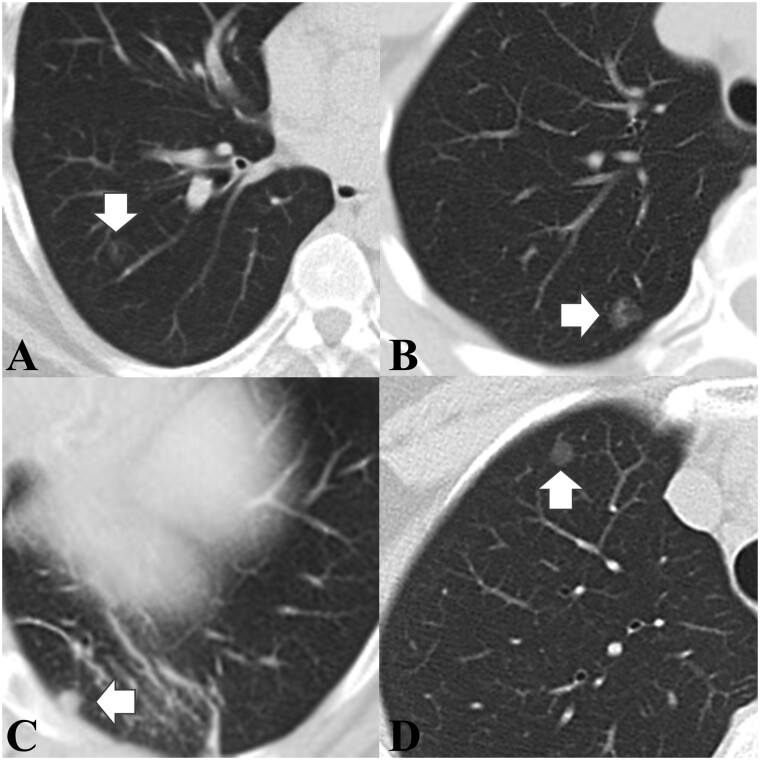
(A and B) Computed tomography (CT) image of solitary pulmonary capillary haemangioma (arrow), which usually presents as (A) a pure ground-glass nodule or (B) part-solid ground-glass nodule similar to the presentation of lepidic-predominant adenocarcinoma of the lung (arrow). (C) Occasionally, solitary pulmonary capillary haemangioma may present as a pure solid nodule. (D) CT image of lepidic-predominant adenocarcinoma (arrow).
